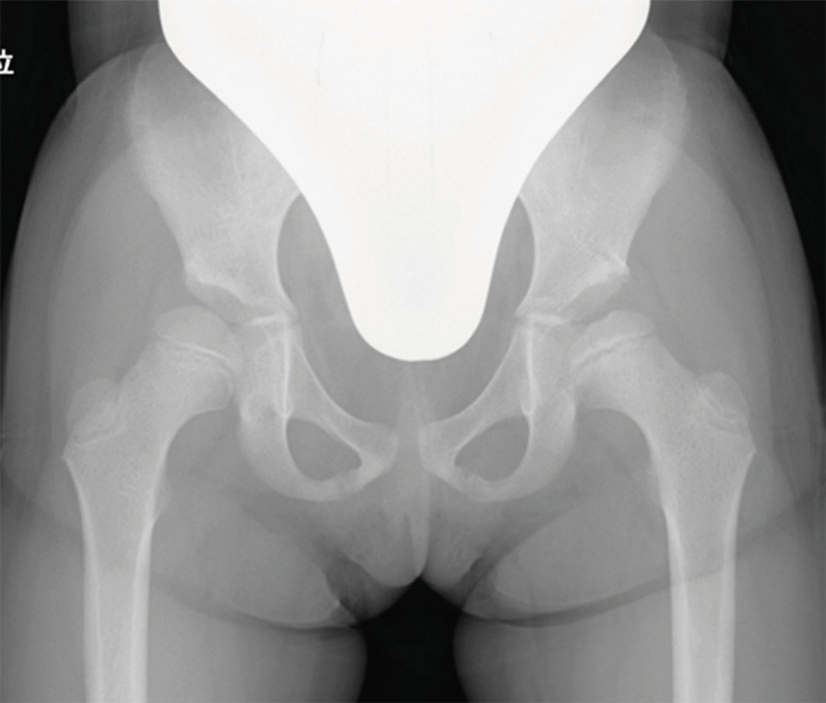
Preoperative radiograph of bilateral hips.
